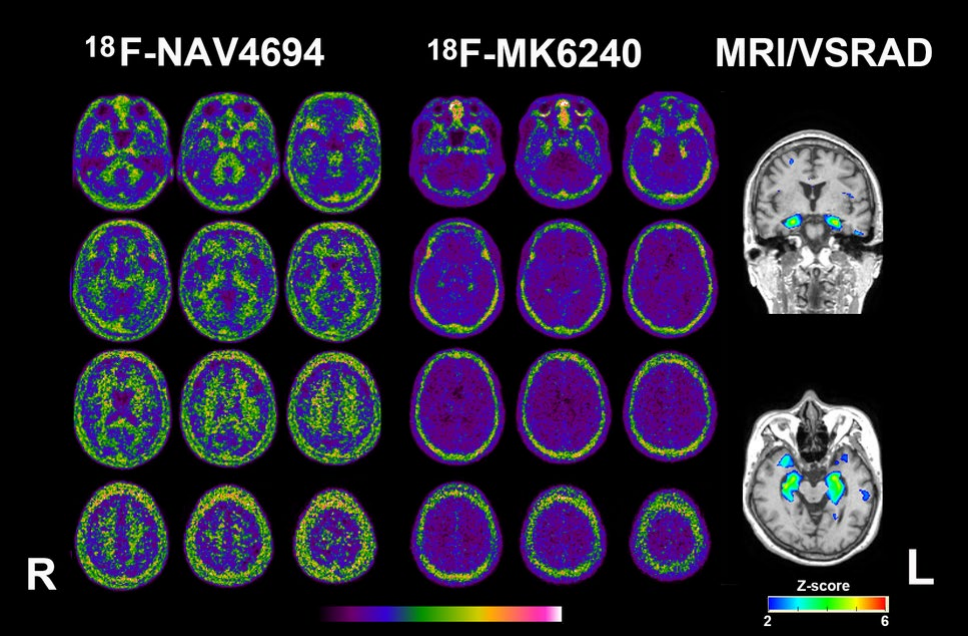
PET and MRI of a woman in her late 60s with primary age related tauopathy (A−T+N+). Amyloid PET is negative. Tau PET Braak stage is determined to be I, as tau deposition is observed only in the bilateral medial temporal regions. The *Z*-score color map, analyzed using VSRAD software, shows significant atrophy in the mesial temporal regions with a *Z*-score of 2 or higher
